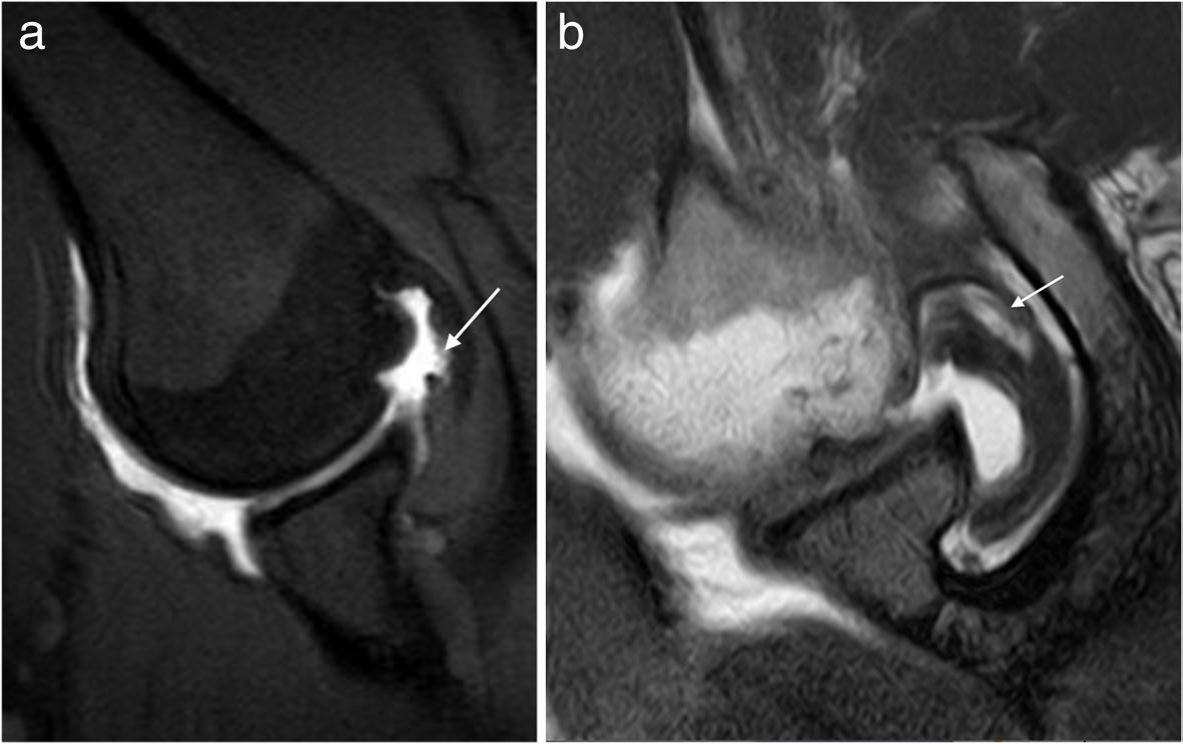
Partial articular-sided tear of the supraspinatus tendon (two different patients). a ABER fat-suppressed PD-weighted arthrographic MRI demonstrate the partial articular tear (arrow). b ABER T1-weighted MRI arthrography reveals an articular tear with horizontal intratendinous extension. Note how the contrast is filling the tear (arrow)
